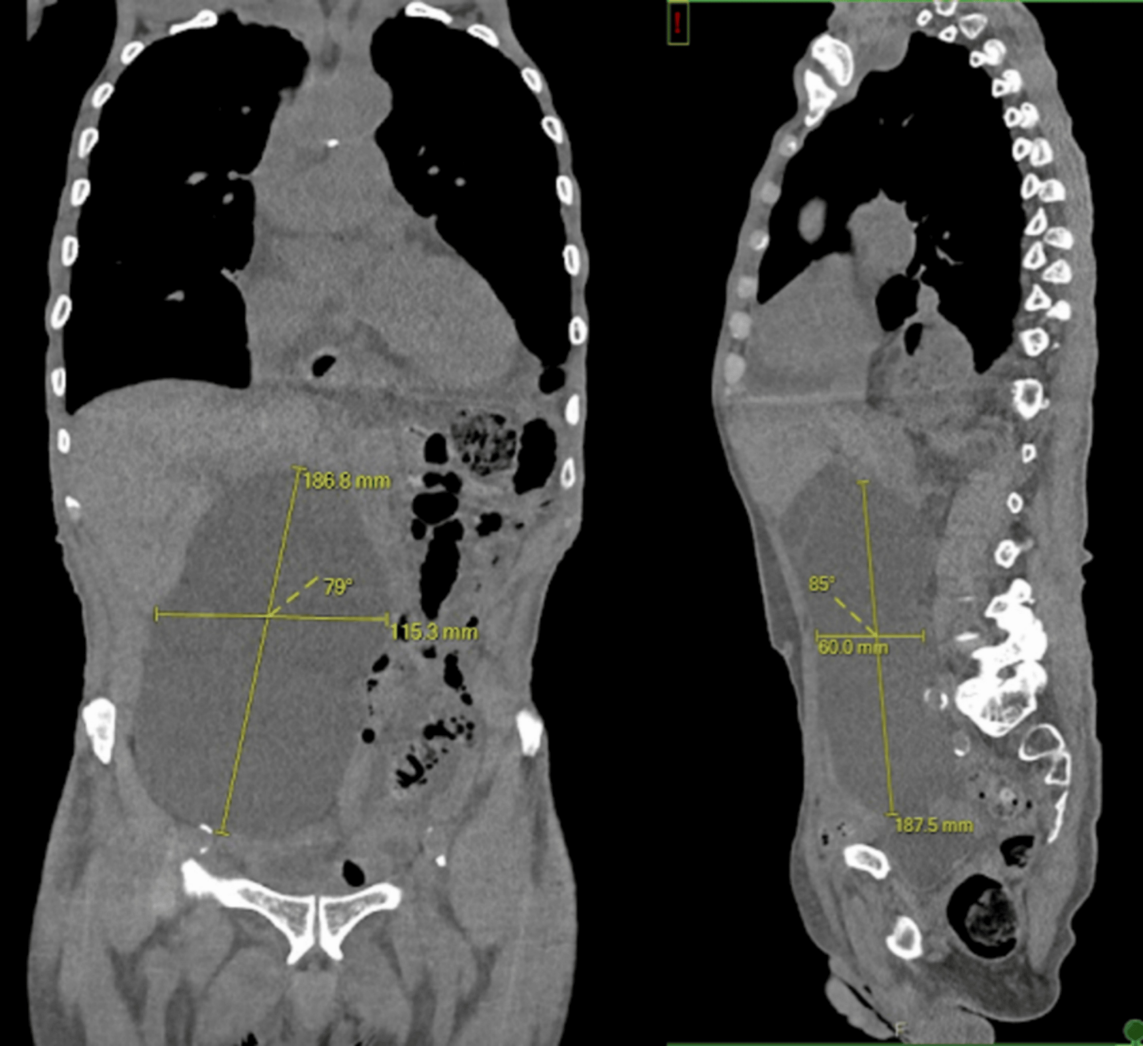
Computed tomography (without contrast) of the abdomen showing the patient's massive renal cyst in coronal and sagittal views. In the largest dimensions, the cyst measures 18.6 cm x 11.5 cm x 6 cm.
